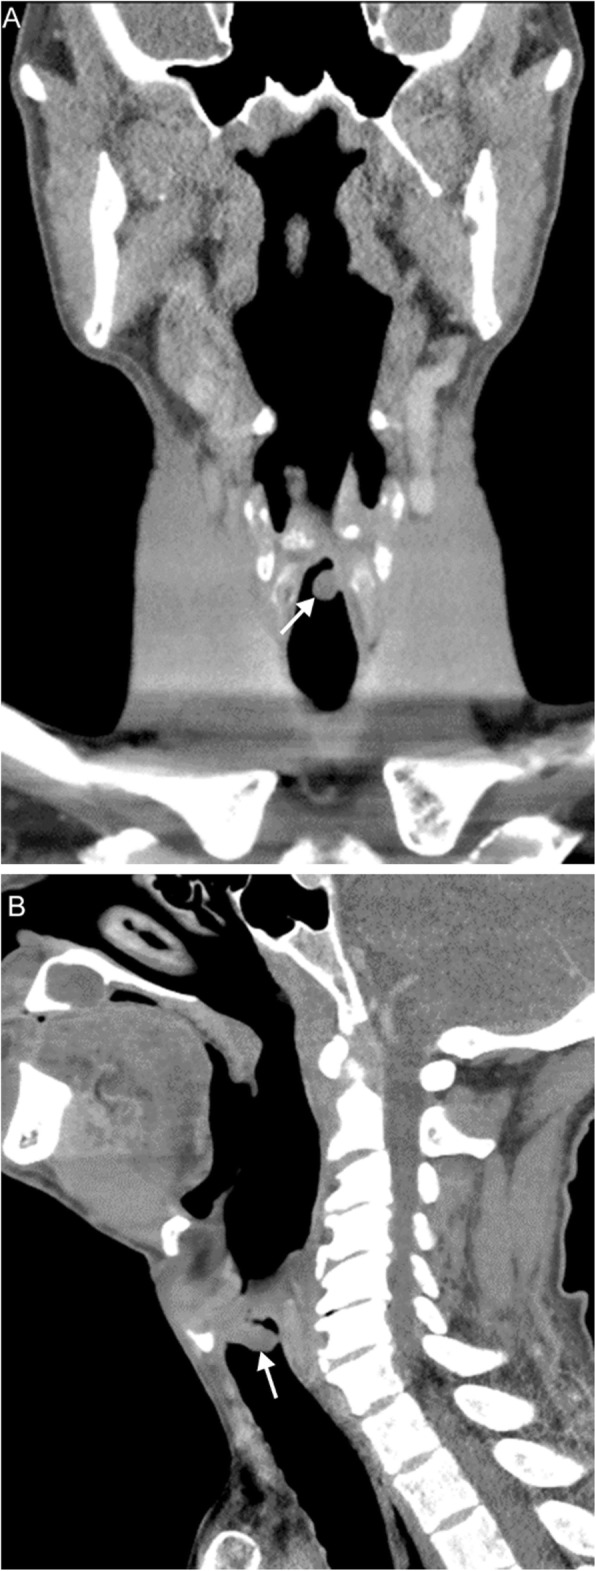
Laryngeal polyp. A 57-year-old man heavy smoker reporting sore throat, hoarseness, and stridor. Coronal (a) and sagittal (b) contrast-enhanced CT images reveal a polypoid lesion arising from the left wall of the larynx (arrows). Laryngoscopy (not shown) revealed a round mass with ball-valve obstruction of the glottis. Subsequent biopsy confirmed a laryngeal polyp
